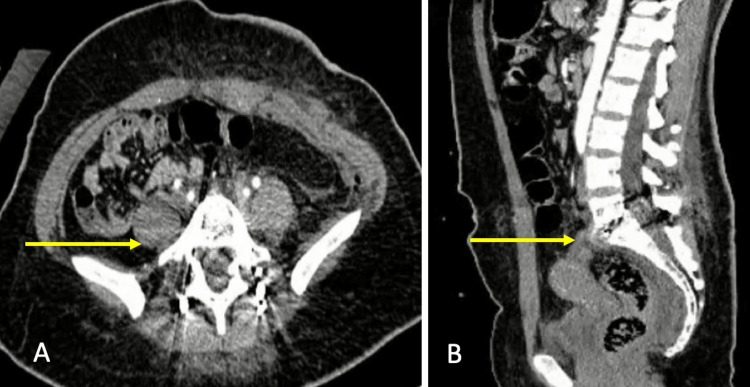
Postoperative imaging studies (A) CT axial section L5-S1 with proper placement of intervertebral cage and transpedicular screws (yellow arrow). (B) Sagittal CT section discarding free fluid and lesions of large vessels in the surgical site (yellow arrow). CT: computed tomography
